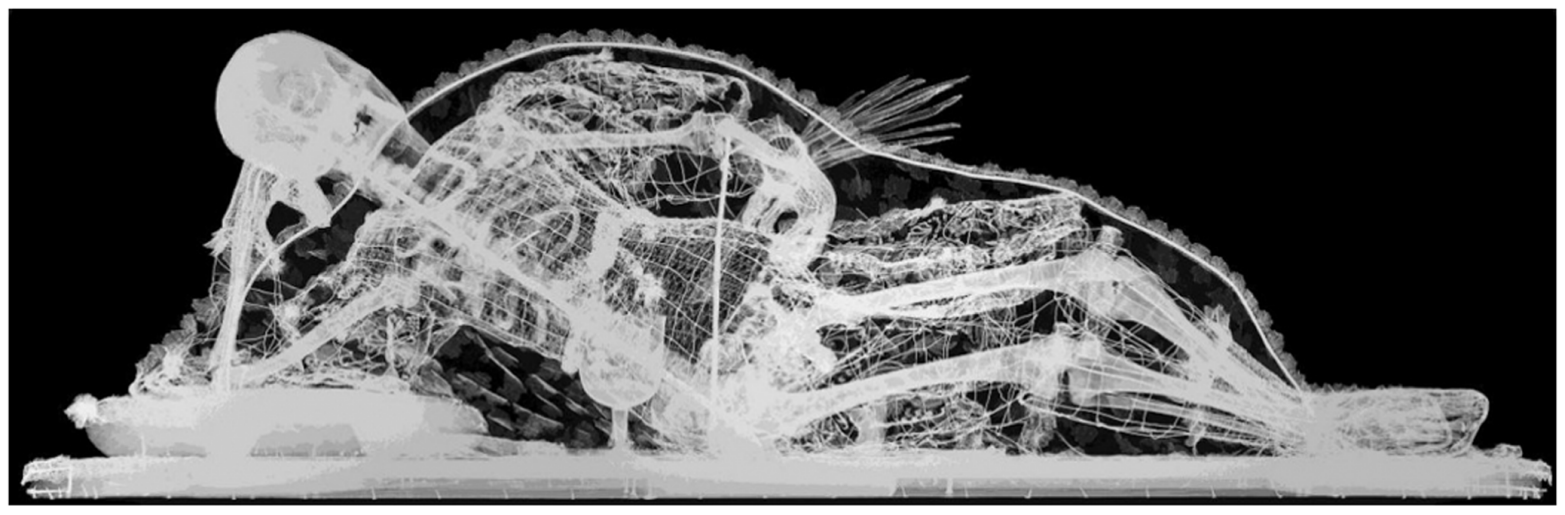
Corposanto* relic-sculpture of St. Aurelius, radiographic view. Human remains (skeletal fragments), metal wire, wood, wax, textile, polychromy, laid paper, and mixed materials. Circa 1789. Cathedral of Porto, Portugal. This figure has been reproduced with permission from: Joana do Carmo Palmeirão, “Imagem-relicário de Santo Aurélio mártir pertencente à Sé Catedral do Porto. Estudo e conservação integrada das relíquias,” MA thesis, Universidade Católica Portuguesa, 2015, 172, fig. 48.
